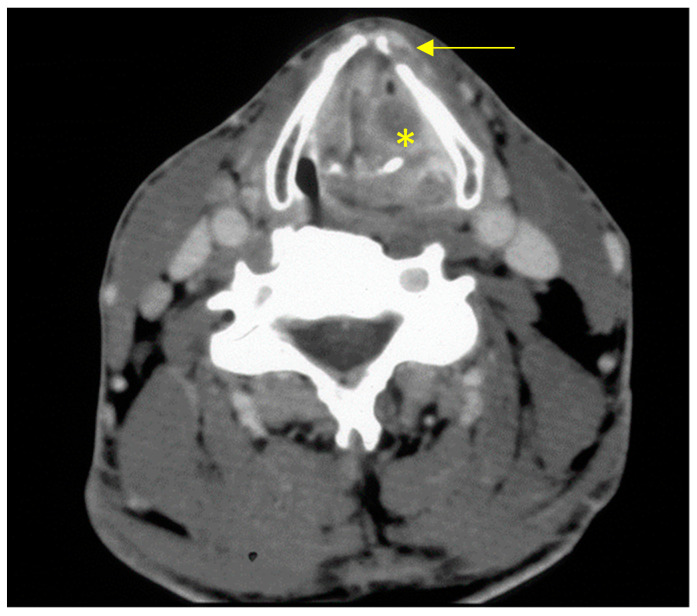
Image from contrast-enhanced CT of the neck from a patient with transglottic cT4a squamous cell carcinoma of the larynx (yellow asterisk = tumour, arrow = cartilage infiltration).
